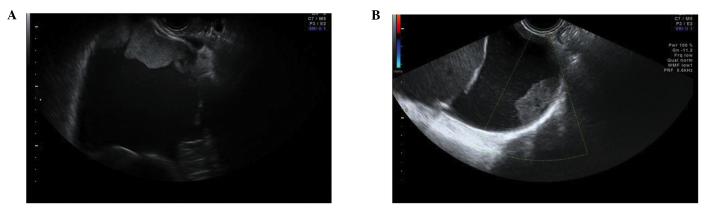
Mucinous endocervical BOT. (A) B mode image. (B) Color Doppler image.
